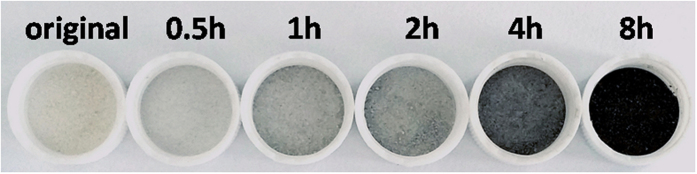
A photo comparing the appearance of original TiO_2_ and ultrasonic treated TiO_2_ for different hours.
